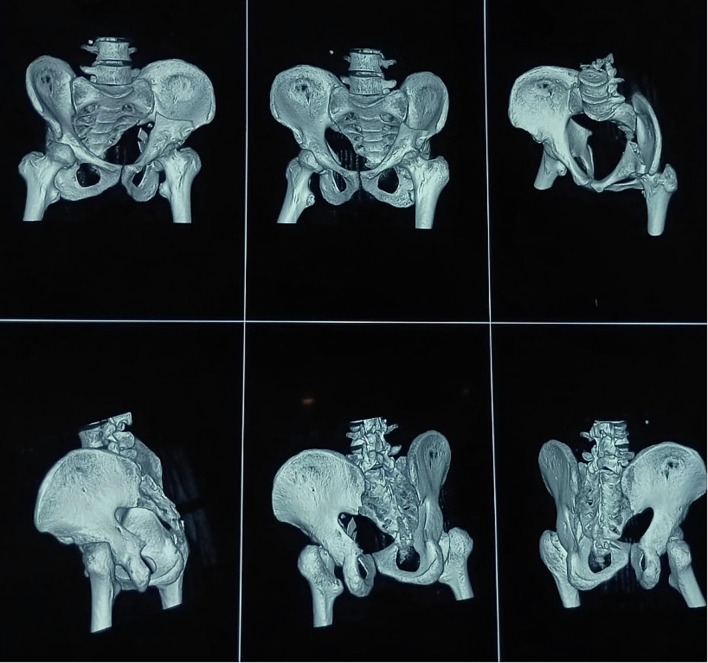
Preoperative CT scan [3D reconstruction].
